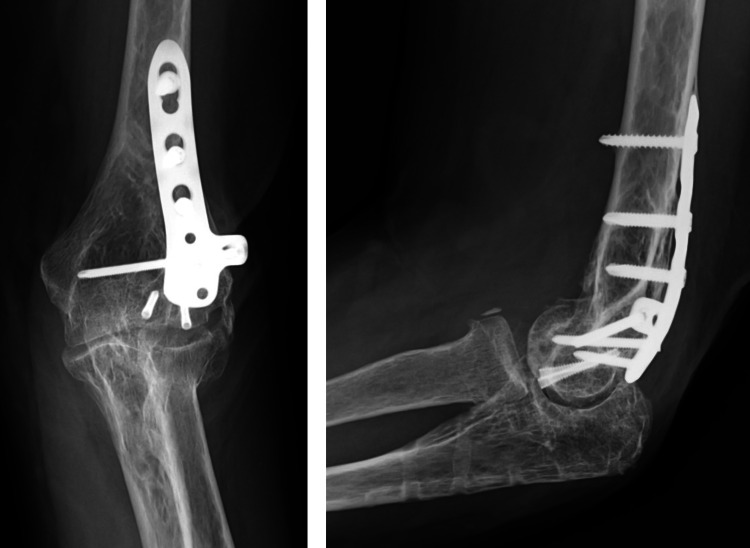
Radiograph at 14 months postoperatively showing that bone union had been achieved. There was no osteonecrosis, but mild arthropathic changes were observed.
